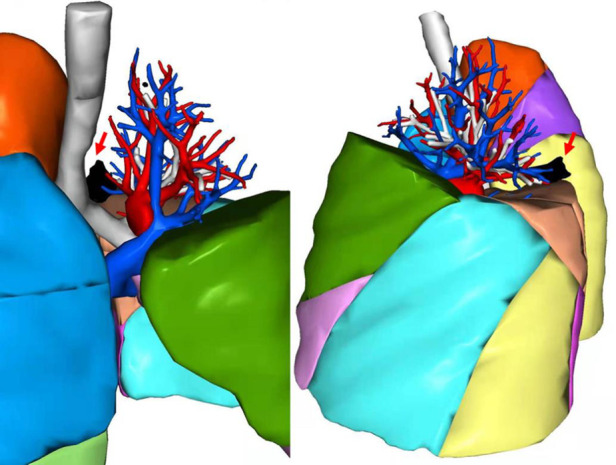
3D reconstruction of CT. Red arrows refers the lung nodule in black.
